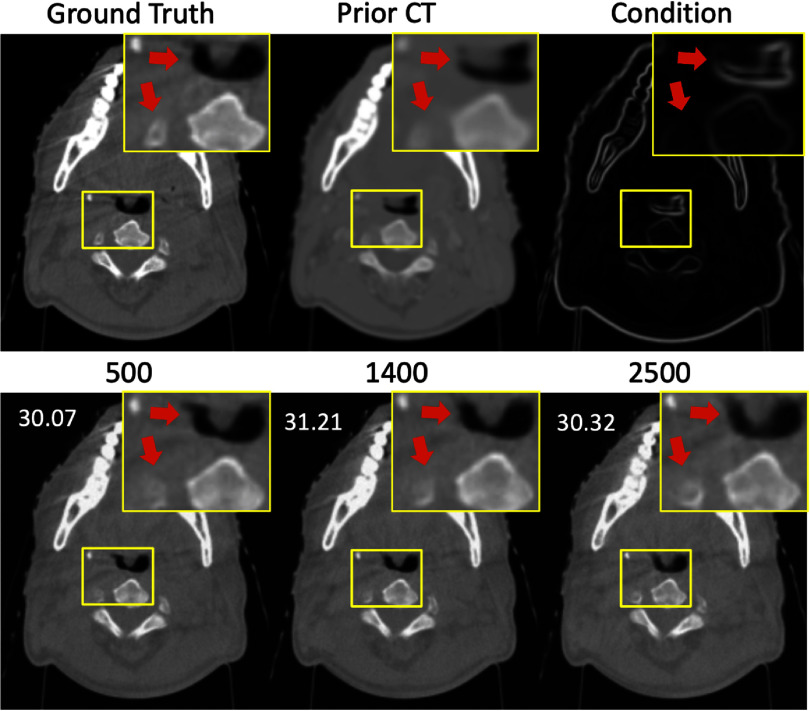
Comparison between reconstructed LA-CBCTs, with the high-frequency prior CT condition dropped at different iteration steps (500, 1400, and 2500) for PFGDM-A. The reconstructions are based on an orthogonal-view 90° scan angle (45° for each view), using a total of 3000 iteration steps. PSNR scores measured against the ‘ground-truth’ fully-sampled CBCT are indicated at the top-left corner.
